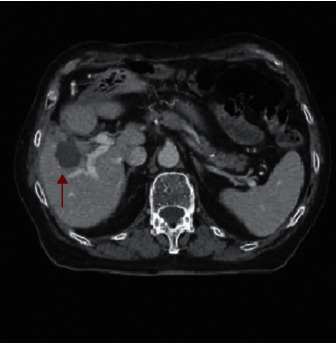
CT imaging of the liver lesion in segment V measuring 39 mm (arrow) that was treated with MWA.
